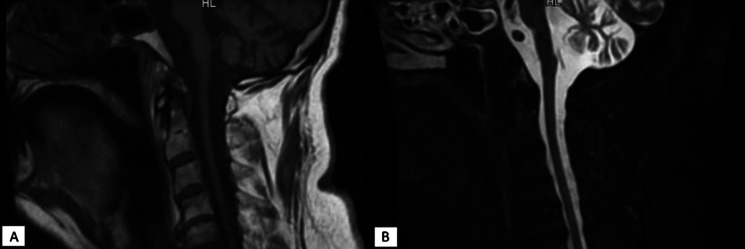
MRI showing marked cerebellar atrophy; A T1 sequence; B STIR sequence
